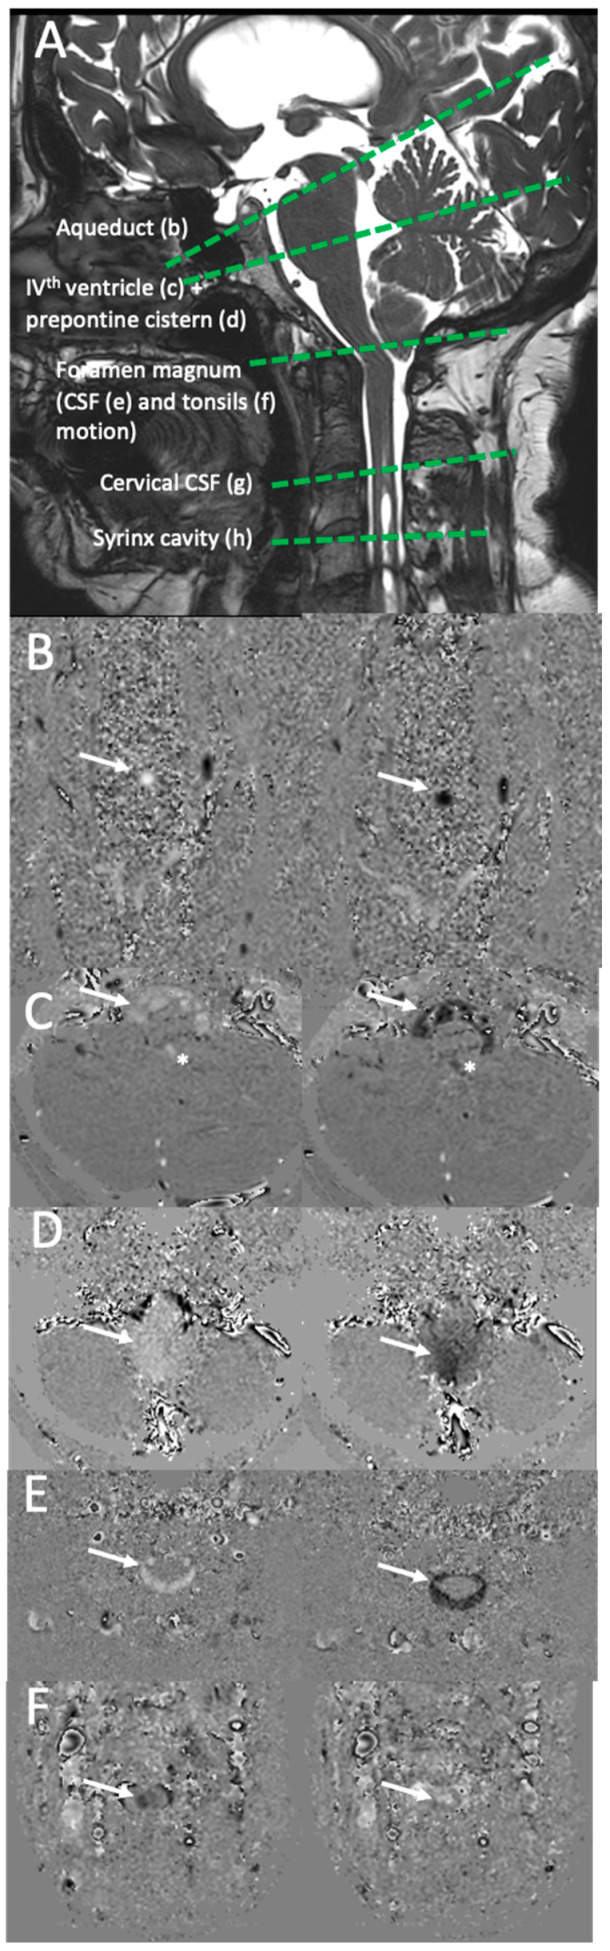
Plane position and phase-contrast images. (A) Cross-sectional plane of phase-contrast MRI acquisitions for the evaluation of craniospinal hydrodynamics. The cross-sectional planes were placed on a BFFE sagittal slice. Aqueduct: slice plane for analysis of cerebrospinal fluid (CSF) dynamics within the aqueduct of Sylvius; PPC (prepontine cistern): slice plane for analysis of subarachnoid CSF dynamics of the prepontine cisterns; FM (foramen magnum): slice plane for analysis of the dynamics of the subarachnoid CSF at the foramen magnum and analysis of the dynamics of the cerebellar tonsils; cervical CSF: analysis of the dynamics of the subarachnoid CSF at the level of the C2C3 disc. (B) Aqueductal phase-contrast acquisition (velocity encoding (Venc = 10 cm/s). The aqueduct is indicated by the arrow. The white signal represents caudocranial displacement of CSF and the black signal represents craniocaudal displacement. These signals represent 2 of the 32 phases of one cardiac cycle. (C) Phase-contrast acquisition at the level of the prepontine cistern (arrow) and IVth ventricle (star) (Venc = 5 cm/s). These signals represent 2 of the 32 phases of one cardiac cycle. (D) Phase-contrast acquisition through the foramen magnum plane (Venc = 2 cm/s). The displacement of the tonsils (arrow) is shown by the white signal (caudocranial displacement) and the black signal (craniocaudal displacement). These signals represent 2 of the 32 phases of one cardiac cycle. (E) Cervical CSF phase-contrast acquisition (Venc = 5 cm/s). The displacement of upper cervical subarachnoidal CSF is shown by the white signal (caudocranial displacement) and the black signal (craniocaudal displacement). These signals represent 2 of the 32 phases of one cardiac cycle. (F) Syrinx phase-contrast acquisition (Venc = 5 cm/s). The displacement of CSF into the syrinx is shown by the white signal (caudocranial displacement) and the black signal (craniocaudal displacement). These signals represent 2 of the 32 phases of one cardiac cycle.
